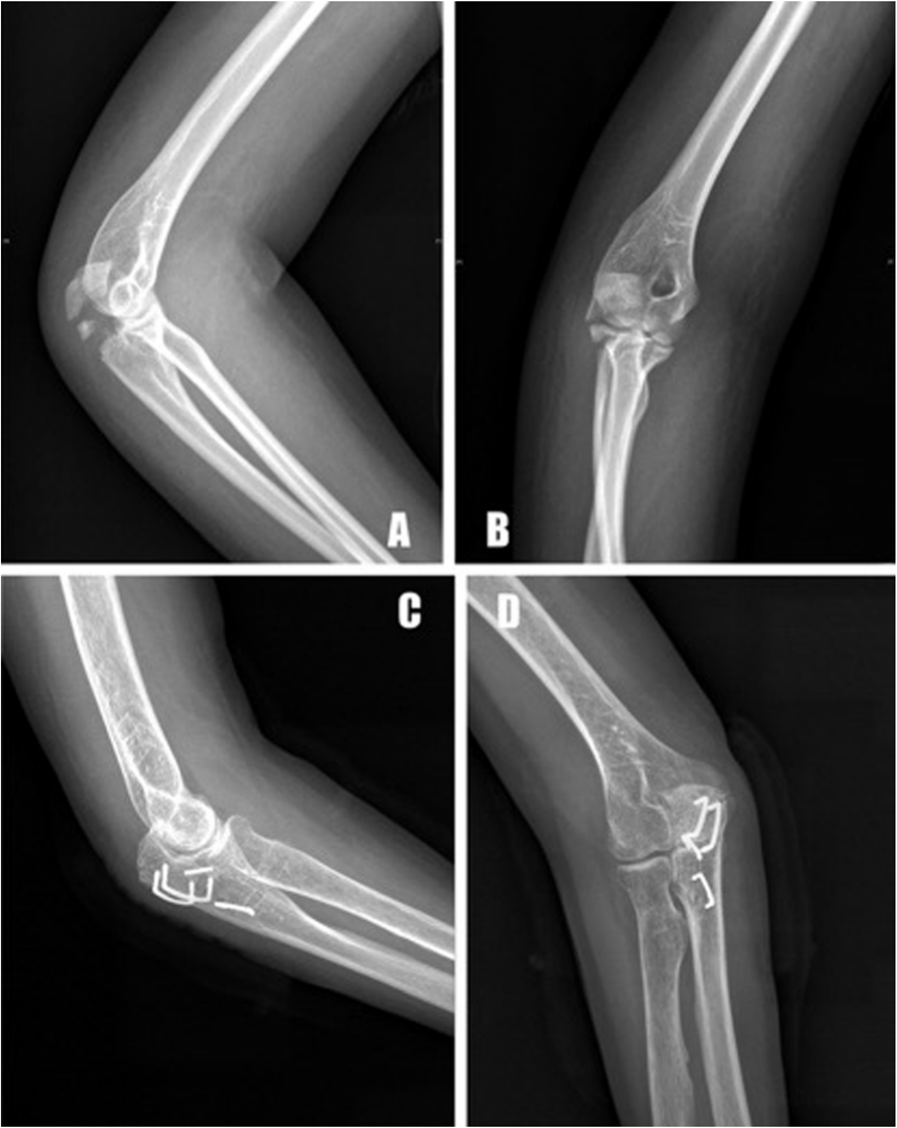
A 55-year-old male patient with commuted olecranon fracture caused by a traffic accident (a, b). Fragments were reduced and fixed with the four ASCs. Eleven months after the surgery, the radiographs showed healed fractures, and the ankle joint surface was anatomically reduced without any gap or step-off (c, d)
